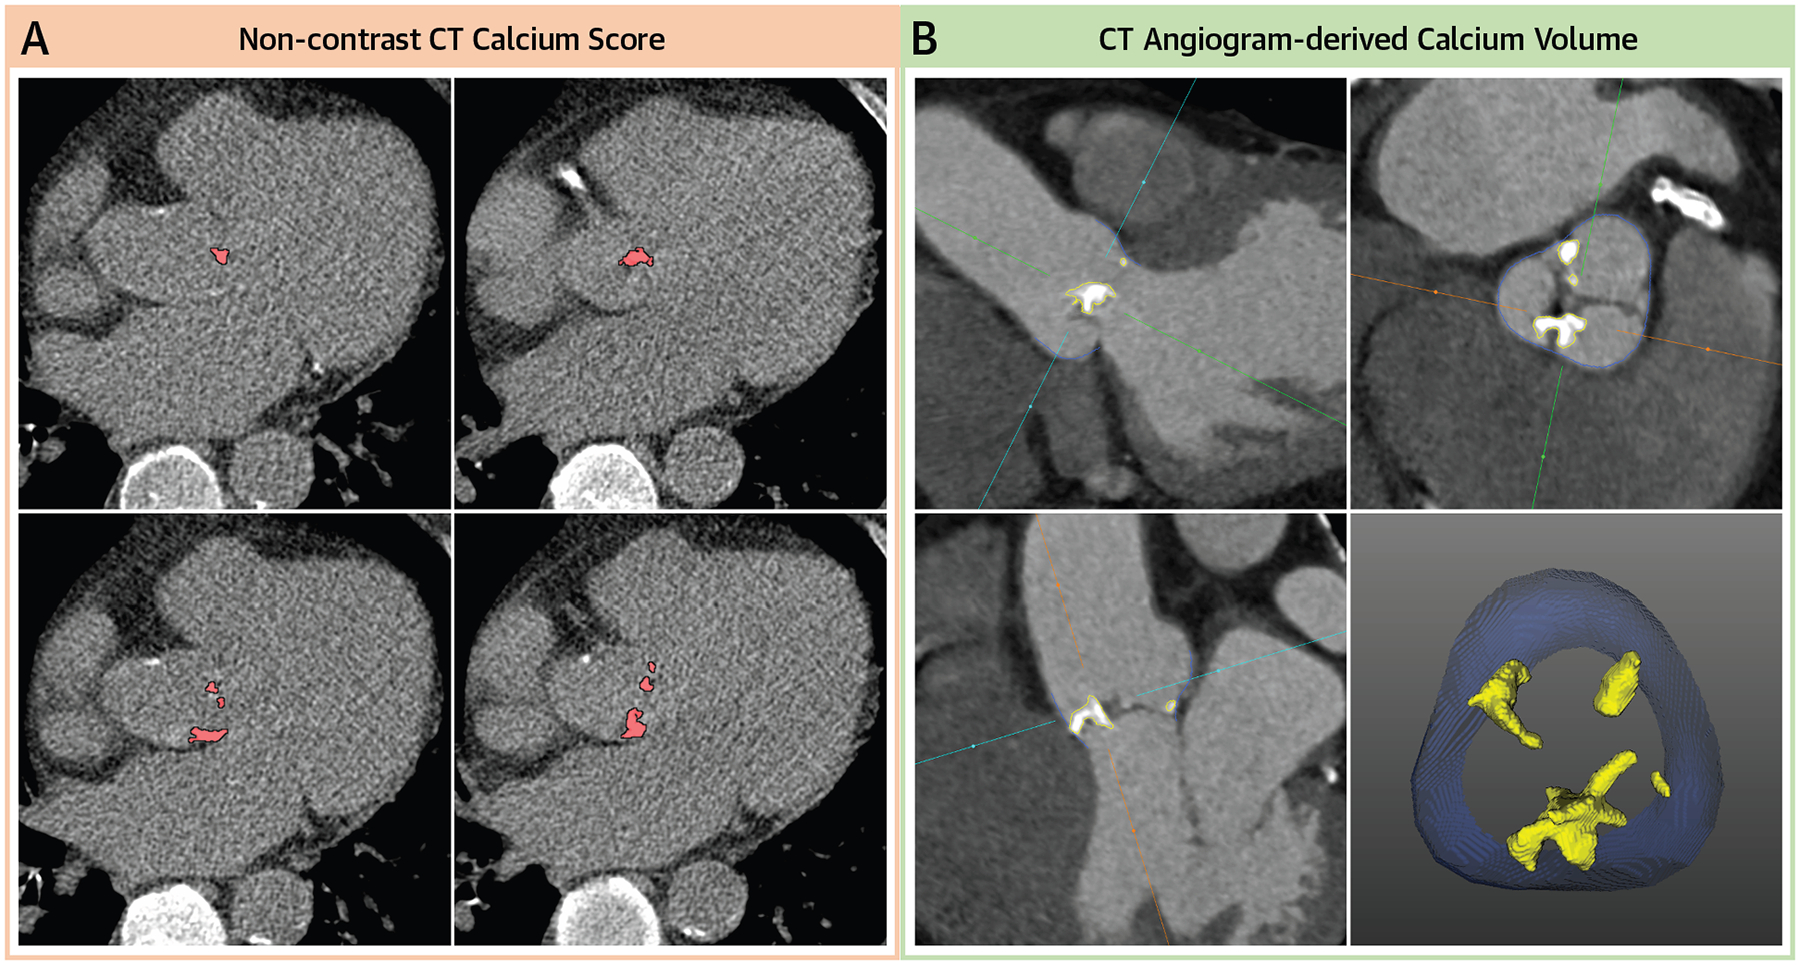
Quantification of Aortic Valve Calcification by CT Aortic valve calcium quantified by (A) noncontrast CT calcium score and (B) CT angiogram–derived calcium volume in a male patient with moderate aortic stenosis on echocardiography, with a calcium score of 1,620 AU and calcium volume indexed to annulus area of 110 mm^3^/cm^2^; both modalities confirm nonsevere disease. AU = Agatston Units; CT = computed tomography.
